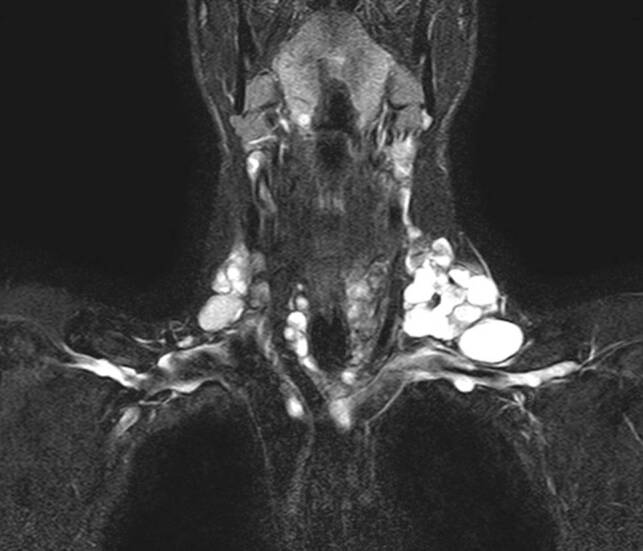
Die Ultraschalldiagnostik für Schilddrüse, Lymphknoten und Nebenschilddrüsen sollte demnach idealerweise auch vom Chirurgen beherrscht werden. Ein positiver Lymphknotennachweis im lateralen Kompartment (Regio IV, V, III, II – einen Überblick zeigt das CT in Abb. 4) lässt dann schon präoperativ eine laterale Halsdissektion einplanen (OP Bild Abb. 5), was sowohl für den Zugangsweg, die geplante Länge der Operation und auch die Wahl des spezialisierten Chirurgen von wesentlicher Bedeutung ist. Schwierig ist speziell die sonographische Lymphknotendiagnostik im zentralen Kompartment (Regio VI) bzw. im oberen mediastinalen Kompartment (Regio VII), die bei routinemäßiger Ultraschalluntersuchung durch Radiologen und Nuklearmediziner nicht immer erfasst wird. Anzumerken ist auch, dass retrotracheale, paraösophageale und vor allem sogenannte retrorecurrente (dorsal des N. laryngeus recurrens) Lymphknoten oft mit der Sonographie nicht einsehbar sind (Abb. 6; [15–17]).
